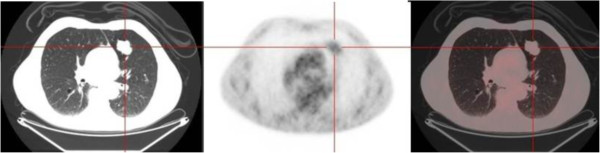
The integrated FDG-PET/CT reveals a large TC (42 mm) demonstrating low uptake (SUVmax 2.2). From left to right: CT, PET and fused image.
